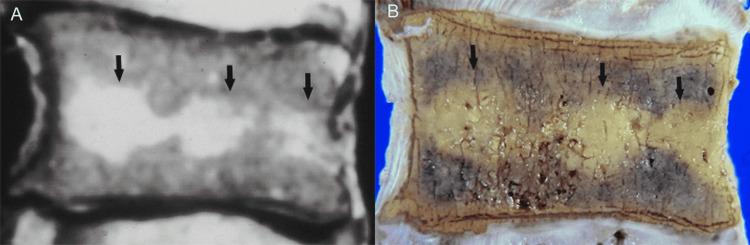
Vertebral marrow conversion. (A) Sagittal T2-weighted MR image shows a high signal intensity zone traversing the center of the vertebral body (arrows). (B) Corresponding anatomic section shows fatty marrow (arrows) in the above-mentioned distribution.
